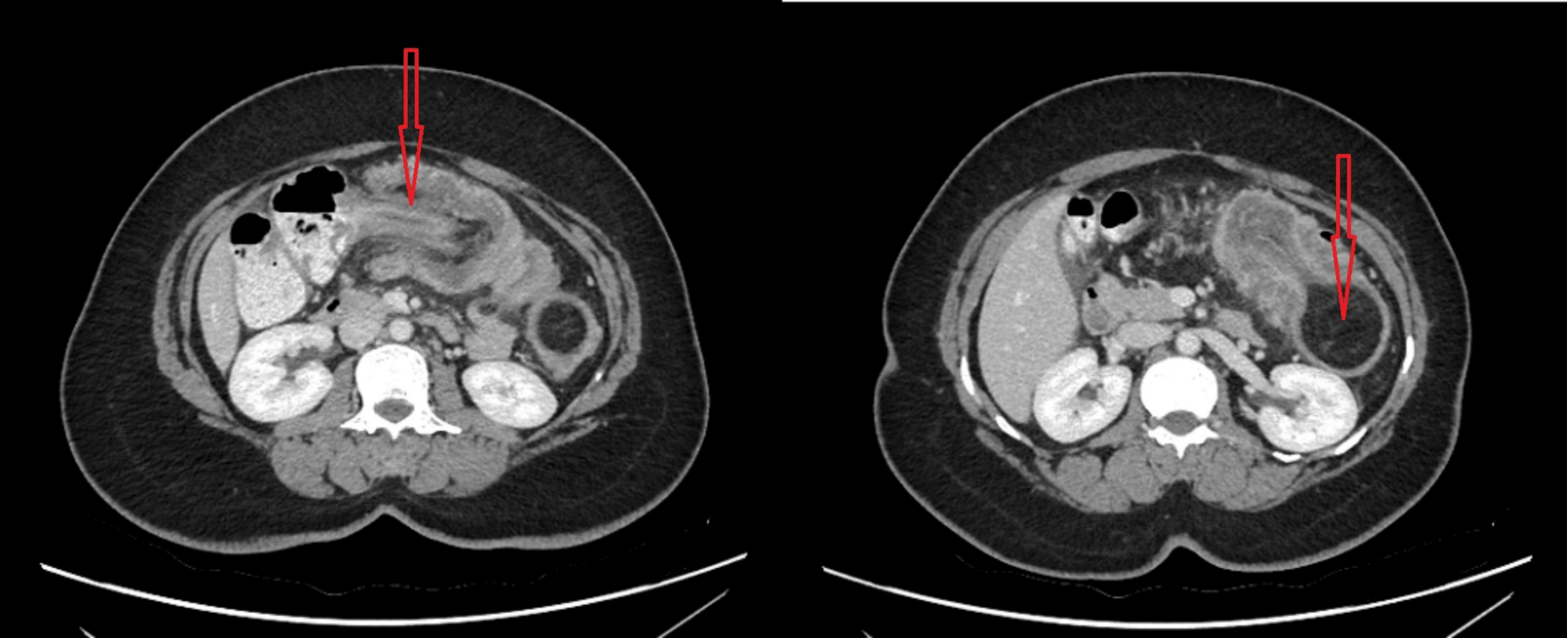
Abdominal CT scan showing colocolic intussusception (A) Intussusception of the transverse colon (red arrow); (B) Distal end of the intussusception showing the colonic lipoma (red arrow)
